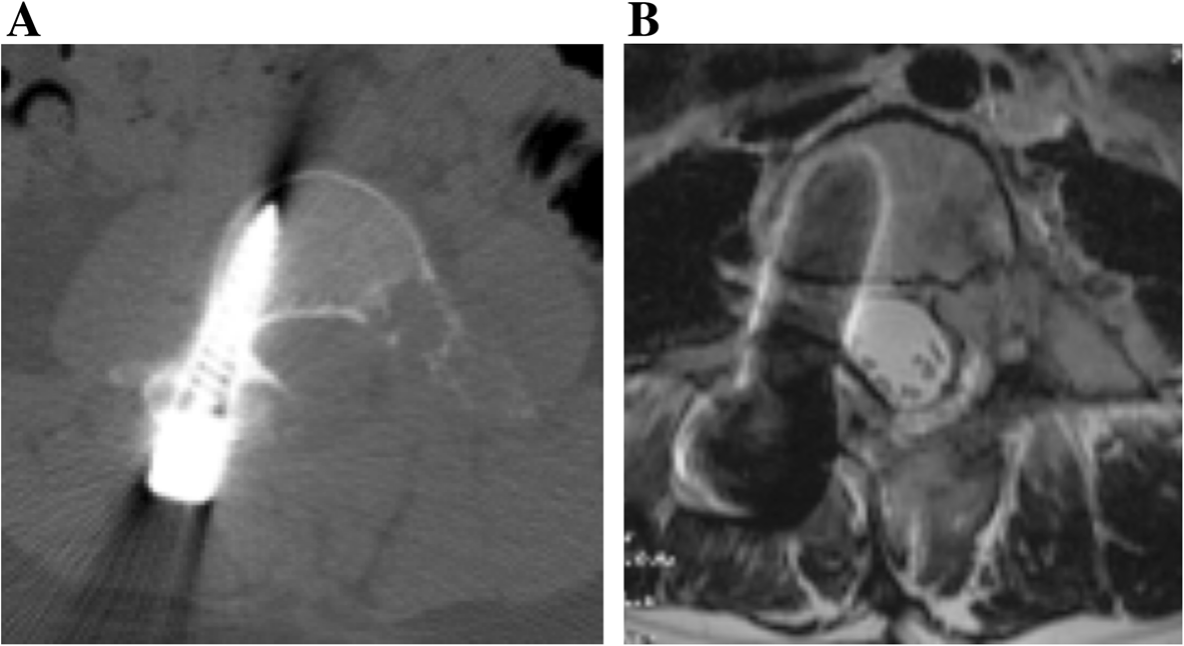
a Axial CT of L4 obtained at approximately 4 months after the initial surgery, at the recurrence of lumbago. Recurrence was suspected. b The same level on T2 axial MRI
